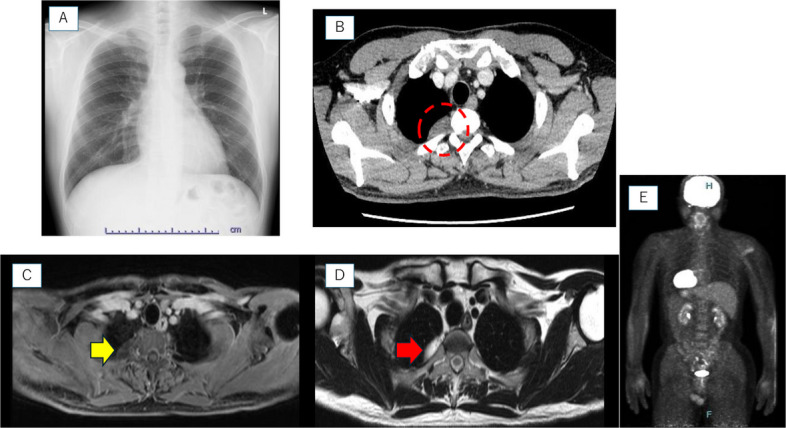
Representative images from the chest examination. A Chest X-ray shows no abnormal shadows. B The contrast-enhanced computed tomography (CT) scan reveals a spindle-shaped lesion with an extrapleural sign adjacent to the vertebral body. C T1-weighted magnetic resonance imaging (MRI) reveals a low-signal lesion (yellow arrow). D T2-weighted MRI shows a high-signal lesion (red arrow). E ^18^F-fluorodeoxyglucose positron emission tomography (FDG-PET)/CT imaging reveals no significant FDG accumulation
